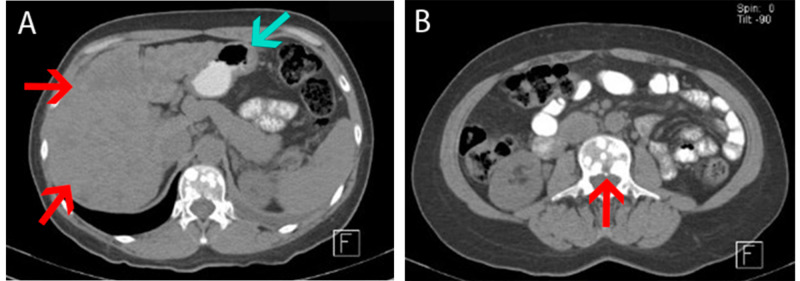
Abdominal CT scan with corresponding images of metastatic lesions A: Multiple hypo- and hyperdense lesions distributed in the liver parenchyma (red arrows), as well as left basal pleural effusion (blue arrow); B: Hyperdense lesion at the L1 level (red arrow) CT: computed tomography
